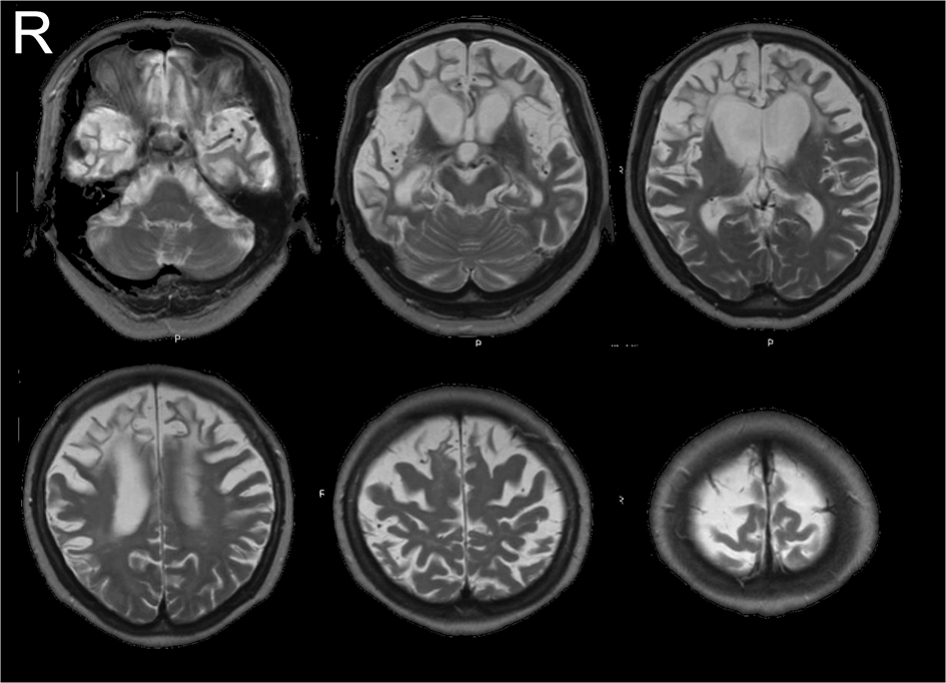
MR images (July 2006). MRI revealed dense atrophy of the bilateral anterior (frontotemporal) lobule, but the posterior (parieto-occipital) lobule was well-preserved.
